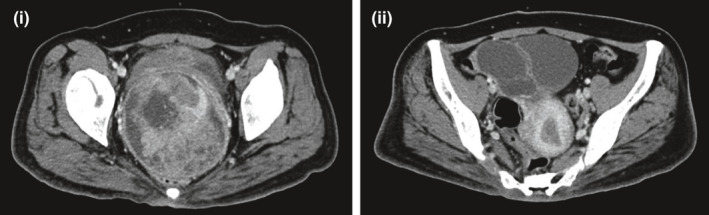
Computed tomography abdomen pelvis scan showing (i) Cervical tumor (ii) Ovarian metastases
